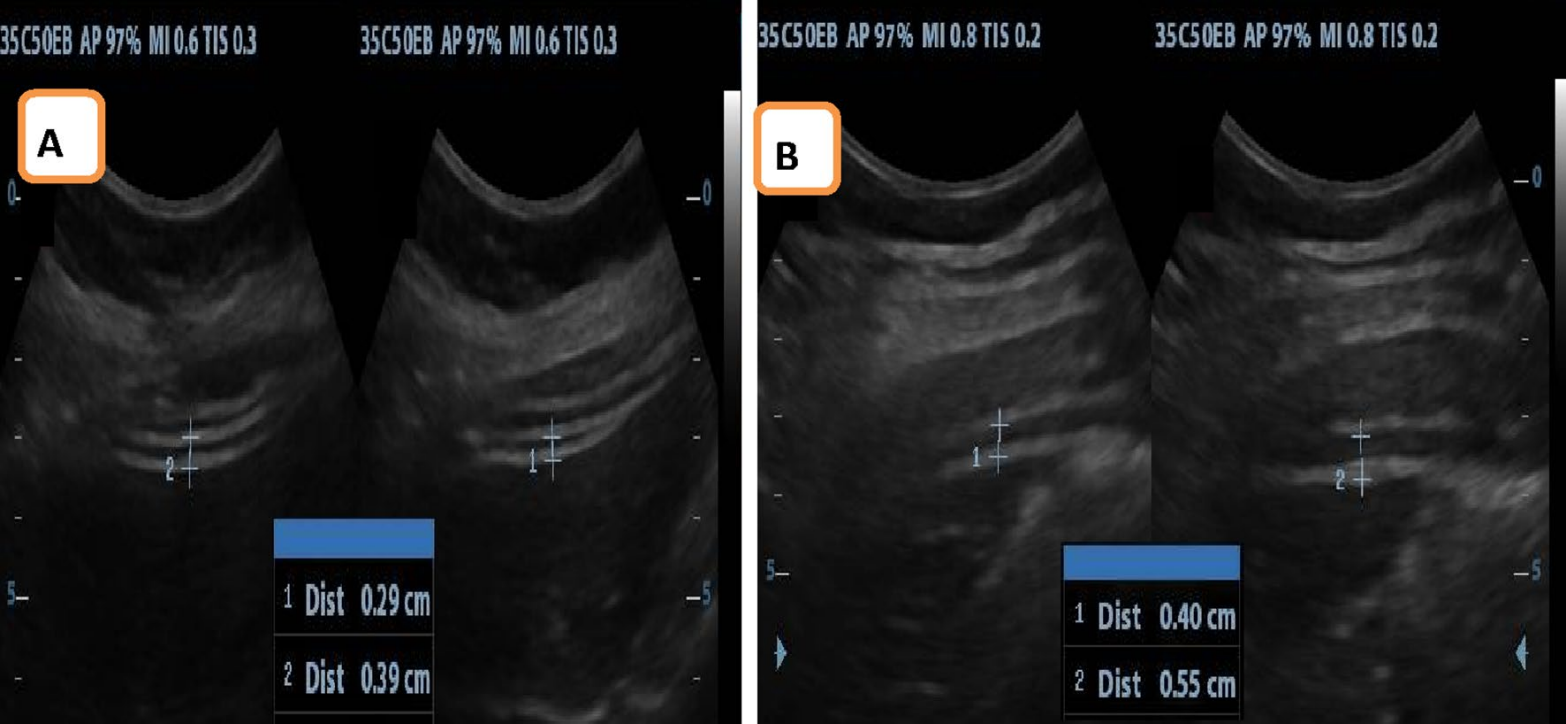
Ultrasound measurement of transversus abdominis activation ratio (A) VL group, (B) control group.
